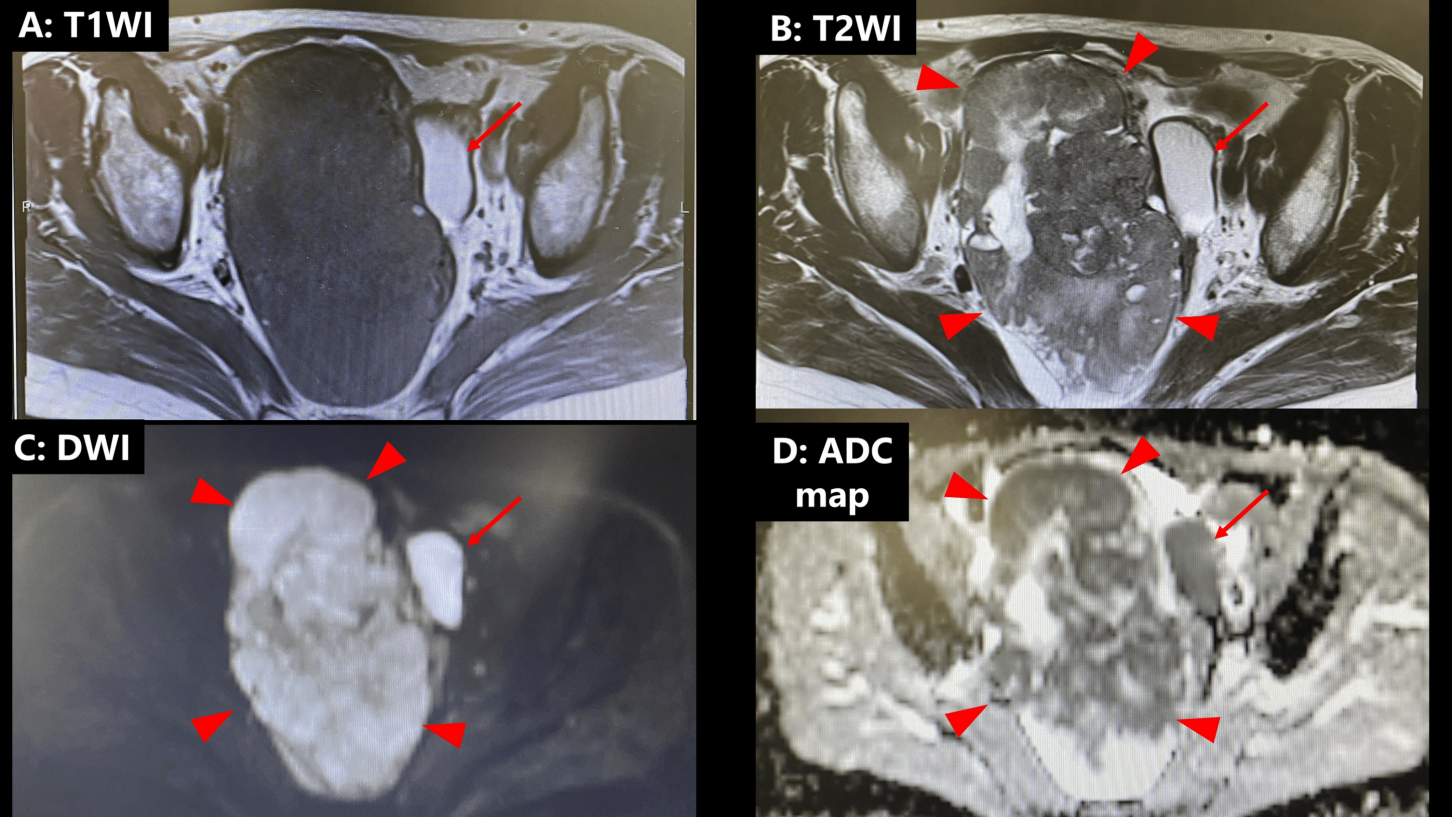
Magnetic resonance imaging features The solid components of the right ovarian tumor show significantly low signal intensity on T2-weighted images (B). Diffusion-weighted images show high signal intensity in the solid region (C). The solid part exhibits a smooth margin. The solid components show low ADC values (0.535×10-3 mm2/s) on the ADC map (arrowhead) (D). The left ovarian cyst shows high signal intensity on both (A) T1- and (B) T2-weighted images and suggests a hemorrhagic ovarian cyst (arrow). Due to the high signal intensity on DWI and low ADC values, along with the presence of a cystic lesion, the diffusion restriction likely reflects hemorrhage (C, D) [9]. T2WI, T2-weighted imaging; DWI, diffusion-weighted imaging; ADC, apparent diffusion coefficient
